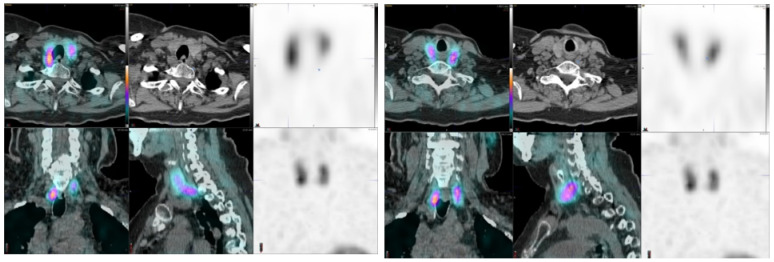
Parathyroid adenoma, on the right (inferior), on the left (superior). This 69-year-old female patient was not operated. Her S-PTH concentration was 113 ng/L and S-Ca 11.0 µg/L. The SUVmax values of the right-sided adenoma were 10.4 (CGZAS) and 7.8 (CGAS), respectively. Visually, this uptake was moderate (2 < TBR < 3) and lesion diameters on CT were 11 mm × 24 mm. The adenoma on the left demonstrated slightly higher SUVmax values: 12 (CGZAS) and 9.3 (CGAS), respectively. Visually this uptake was weaker, but moderate (2 < TBR < 3) and the lesion diameters on CT were 17 mm × 38 mm.
